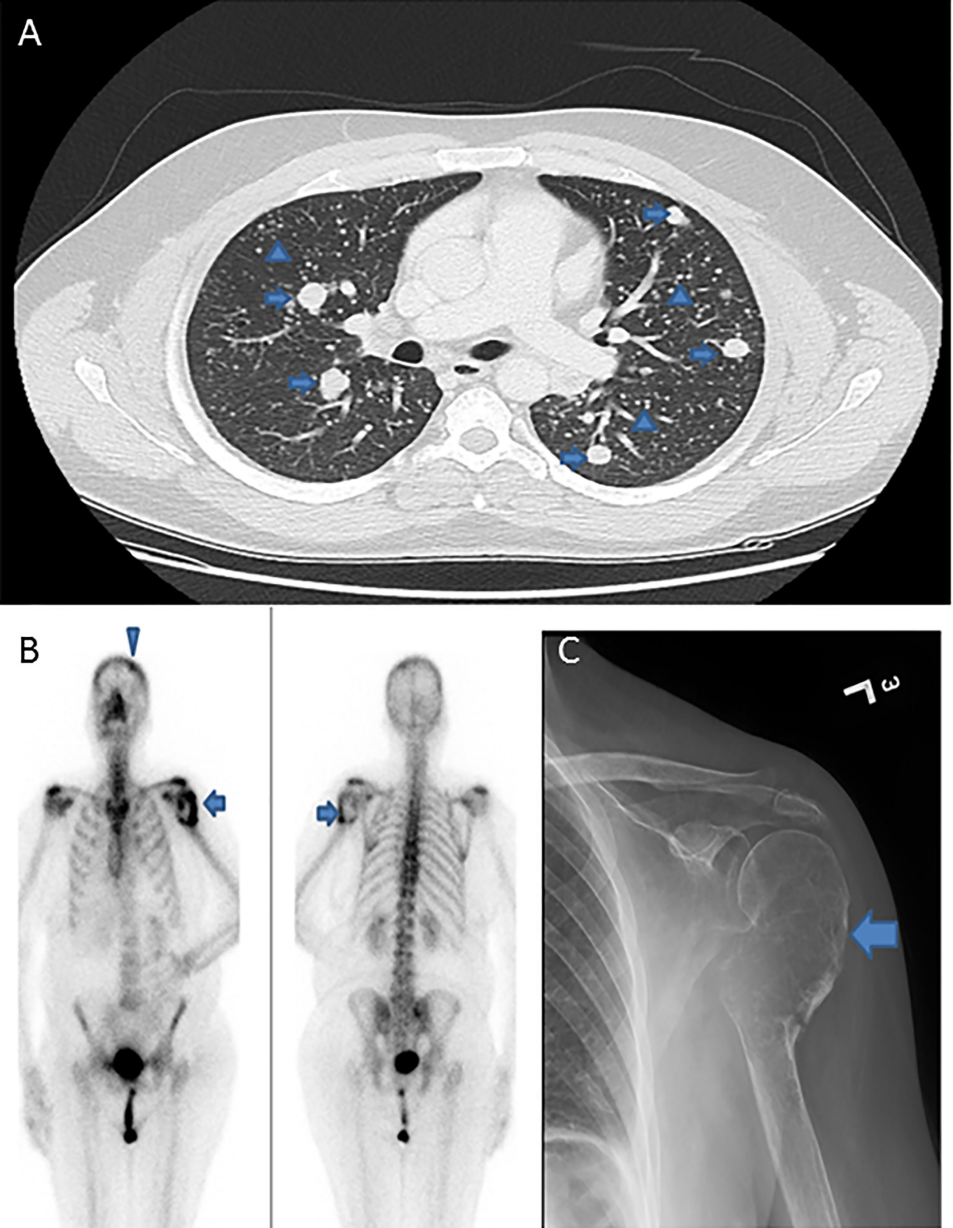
Radiologic images of distant metastasis in papillary thyroid microcarcinoma cases. (A) Computed tomography of the lung showing extensive pulmonary metastases with classic canon-ball metastases (arrows) and miliary metastatic nodules (arrow heads). (B) Positron emission tomography (PET) scan and (C) X-ray, showing expansile lytic metastatic lesion in the left humerus (arrows) with pathologic fracture related to metastasis. PET scan also shows a metastatic focus in the skull bone (arrow head).
